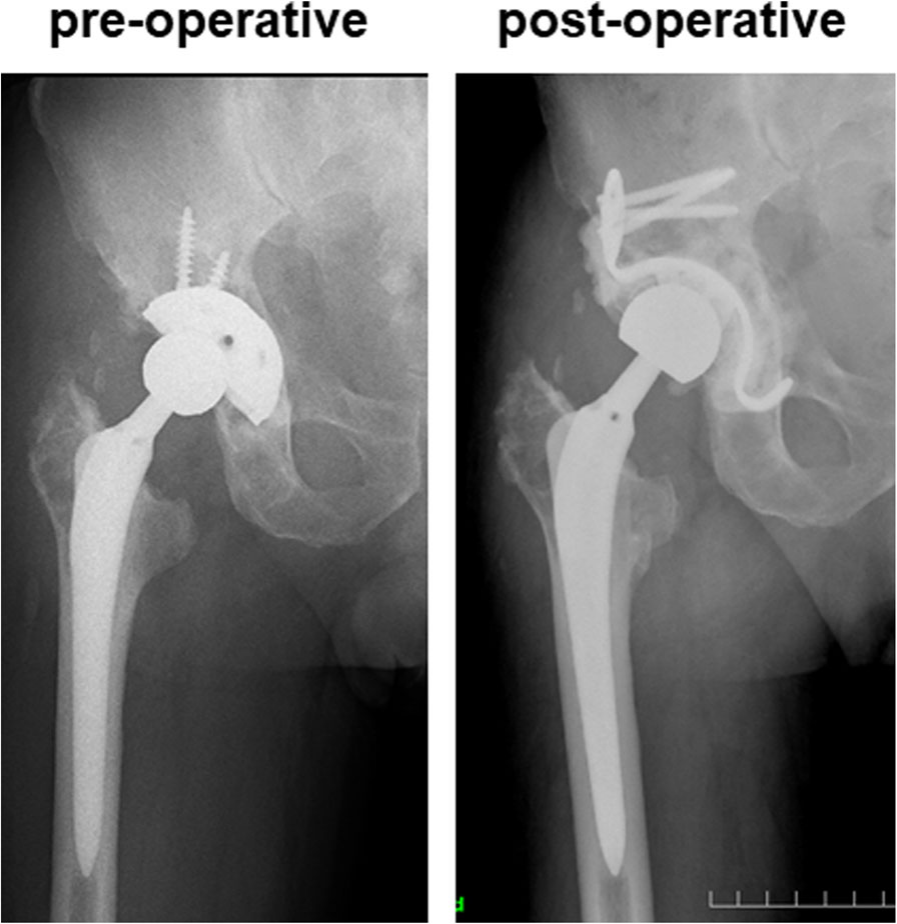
Representative case of preoperative and postoperative X-rays
